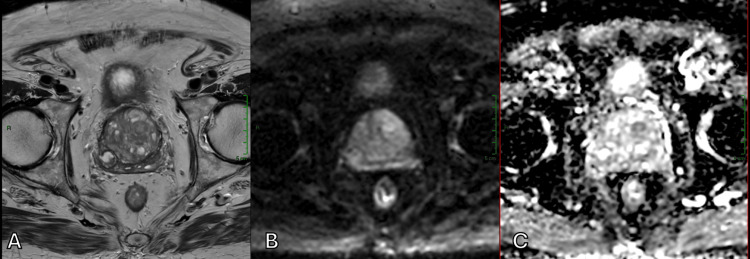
Appearance of “disrupted organized chaos” by a hypointense lesion on T2WI, which shows restricted diffusion with high signal on DWI and reduced values on the ADC map, located in the left transition zone (A) Axial T2WI. (B) Axial DWI. (C) Axial ADC map. ADC, apparent diffusion coefficient; DWI, diffusion-weighted imaging; T2WI, T2-weighted imaging
